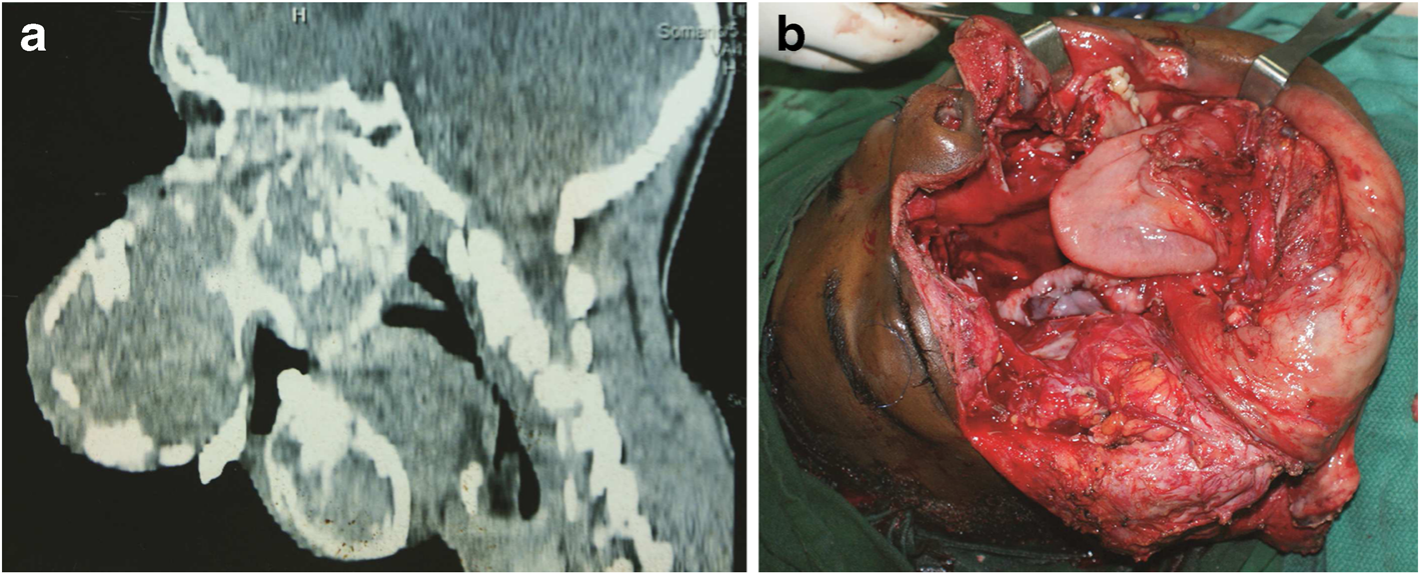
CT scans. (a) CT scan showing large synchronous maxillary and mandibular tumors. (b) Axial CT scan showing extensive maxillary tumor.
